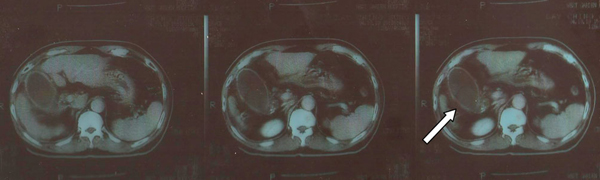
Computed tomography findings. Gall stones, diffusely thickened gall bladder wall with increased contrast enhancement, and a hypodense mass lesion (arrow) without contrast enhancement are noted in the gall bladder lumen.
